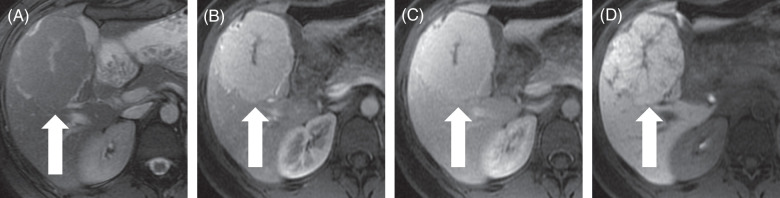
Focal nodular hyperplasia—Characteristic features on MRI with contrast. A 22-year-old woman with focal nodular hyperplasia (arrow) undergoes MRI with hepatobiliary contrast. (A) Axial T2-weighted images show a lesion with T2 iso- to minimal hyperintensity. (B) After injection of contrast, axial T1-weighted images in the arterial phase show that the lesion has homogeneous hyperenhancement with the exception of a central, stellate area known as the central scar. (C) In the portal venous phase, the enhancement persists slightly above the liver. (D) In the hepatobiliary phase, the mass is mostly iso- to hyperintense compared to the uninvolved liver; the central scar is most pronounced in this phase.
